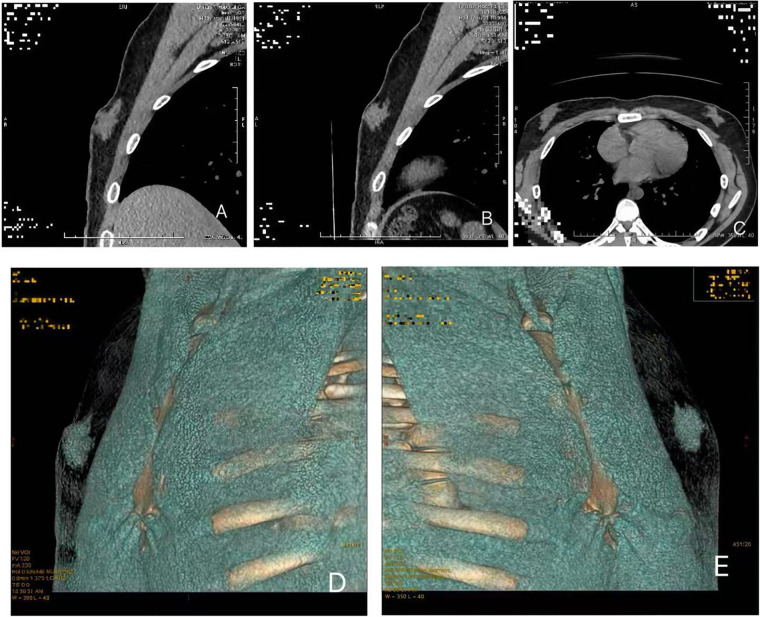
A 34-year-old male patient shown preoperative low-dose CT images at (A) left sagittal, (B) right sagittal and (C) horizontal view; and preoperative at (D) left 3D reconstruction and (E) right 3D reconstruction views.
